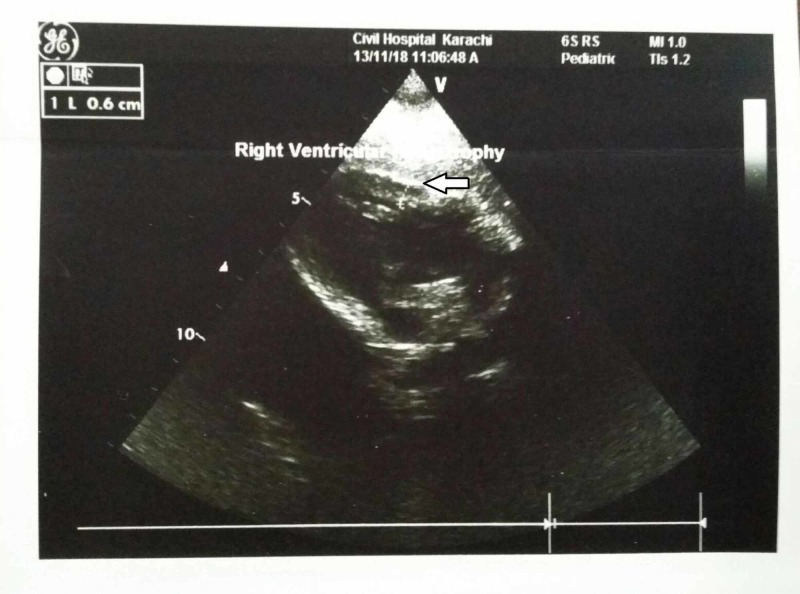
Transthoracic echocardiogram showing right ventricular hypertrophy
